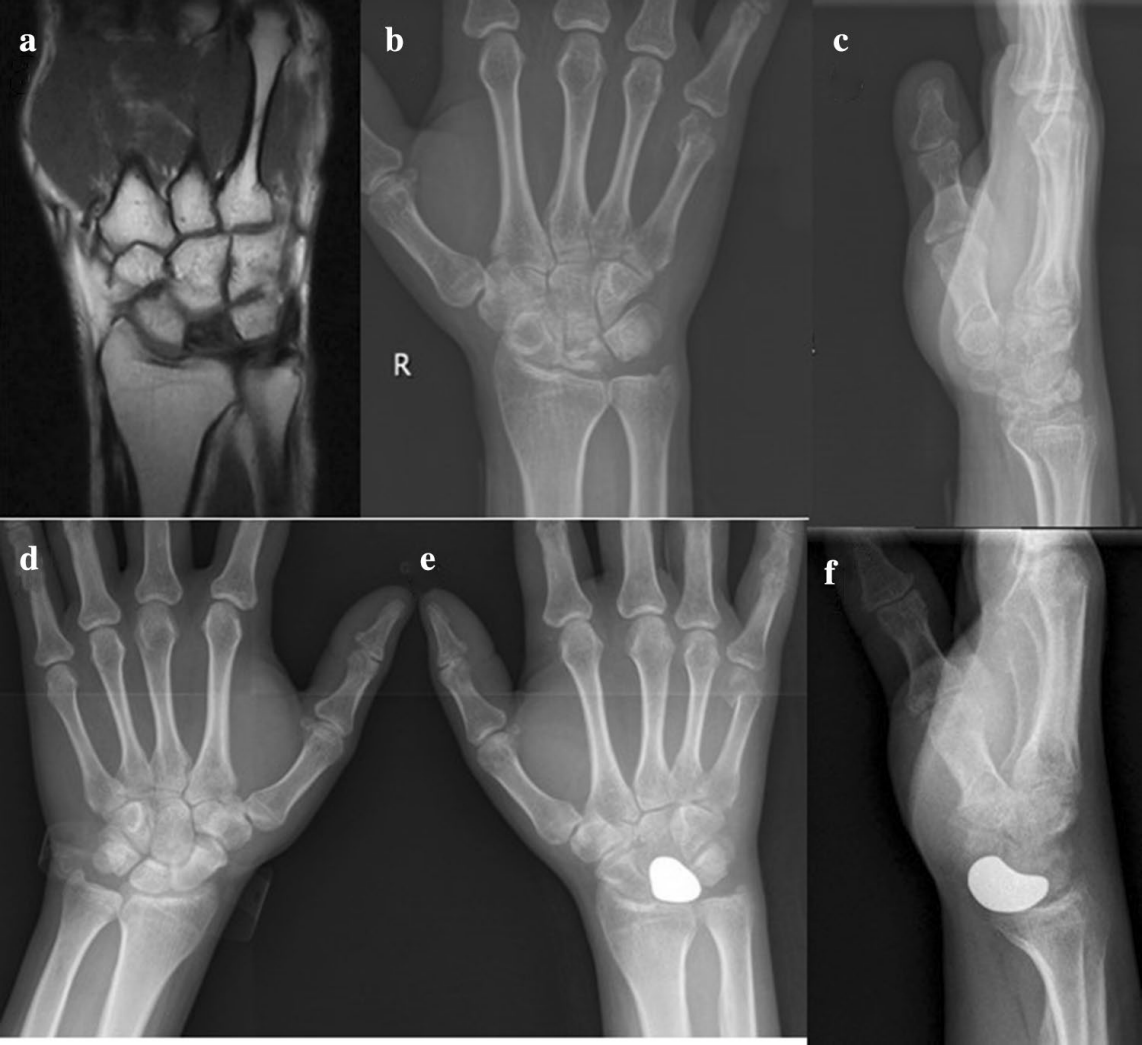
Preoperative MRI and X-rays showed that the lunate density was uneven and the height and width of lunate were collapsed (a–c); the intact wrist X-ray (d); the 3D printing lunate prosthesis was placed in the original anatomic position (e, f)
